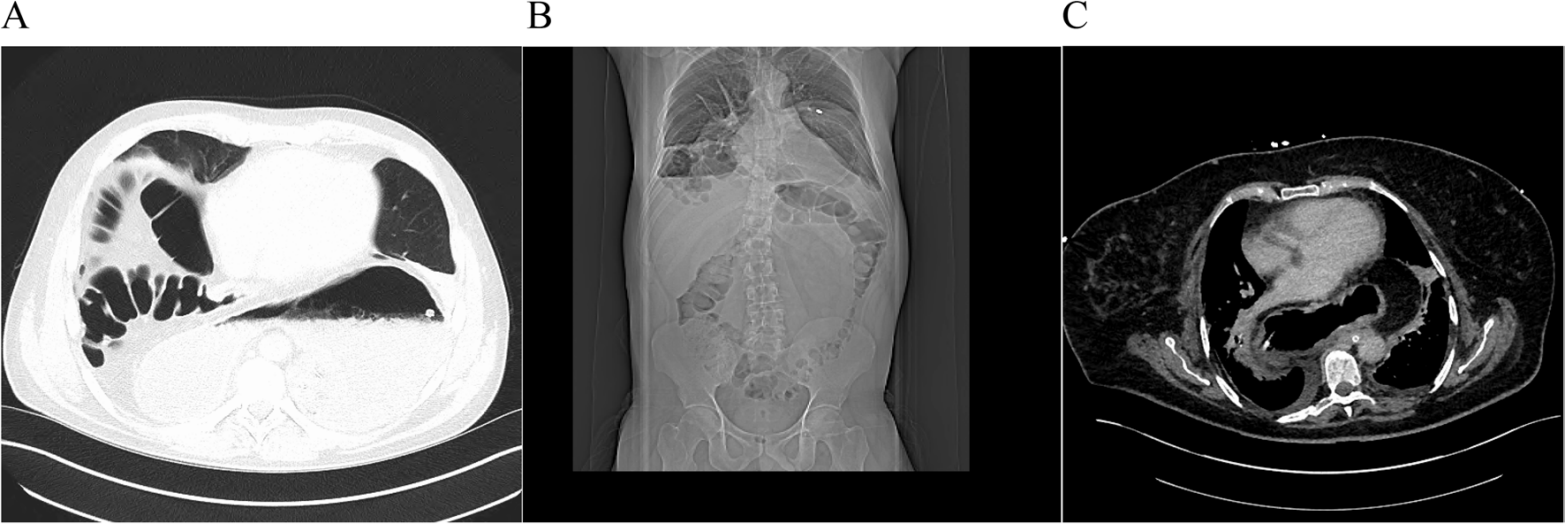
CT images of giant, type IV hernias. The figure shows pre-operative CT images of patients in our review, with type IV giant hiatal hernias of ≥ 10cm in length, with stomach and colon in the thorax
